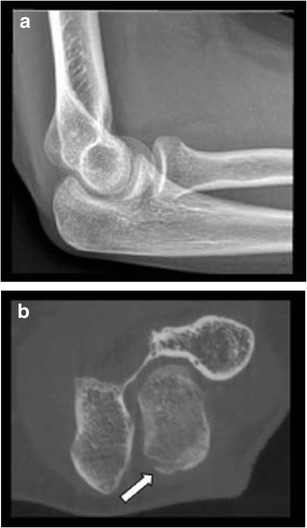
Occult olecranon fracture. a. Lateral view CR of the elbow shows no evidence of fracture. b. Axial CBCT reformatted image reveals subtle cortical disruption and a small adjacent bone fragment at the posterior aspect of the olecranon process
